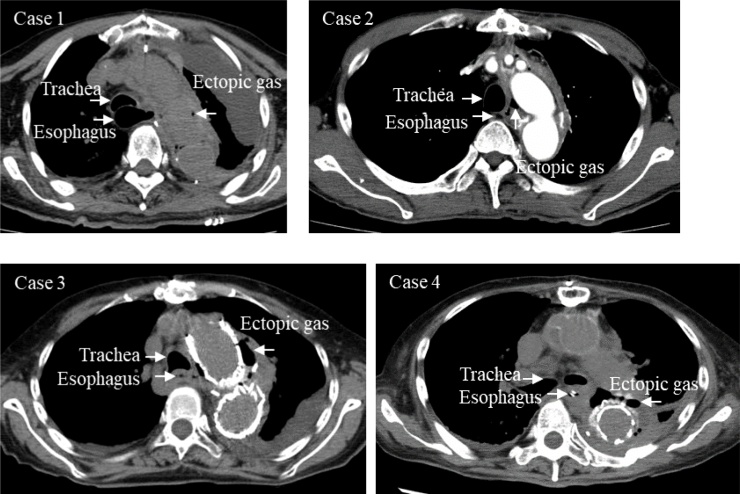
Computed tomography of the chest revealed ectopic gas around the prosthesis, which appears continuous from the esophagus.
